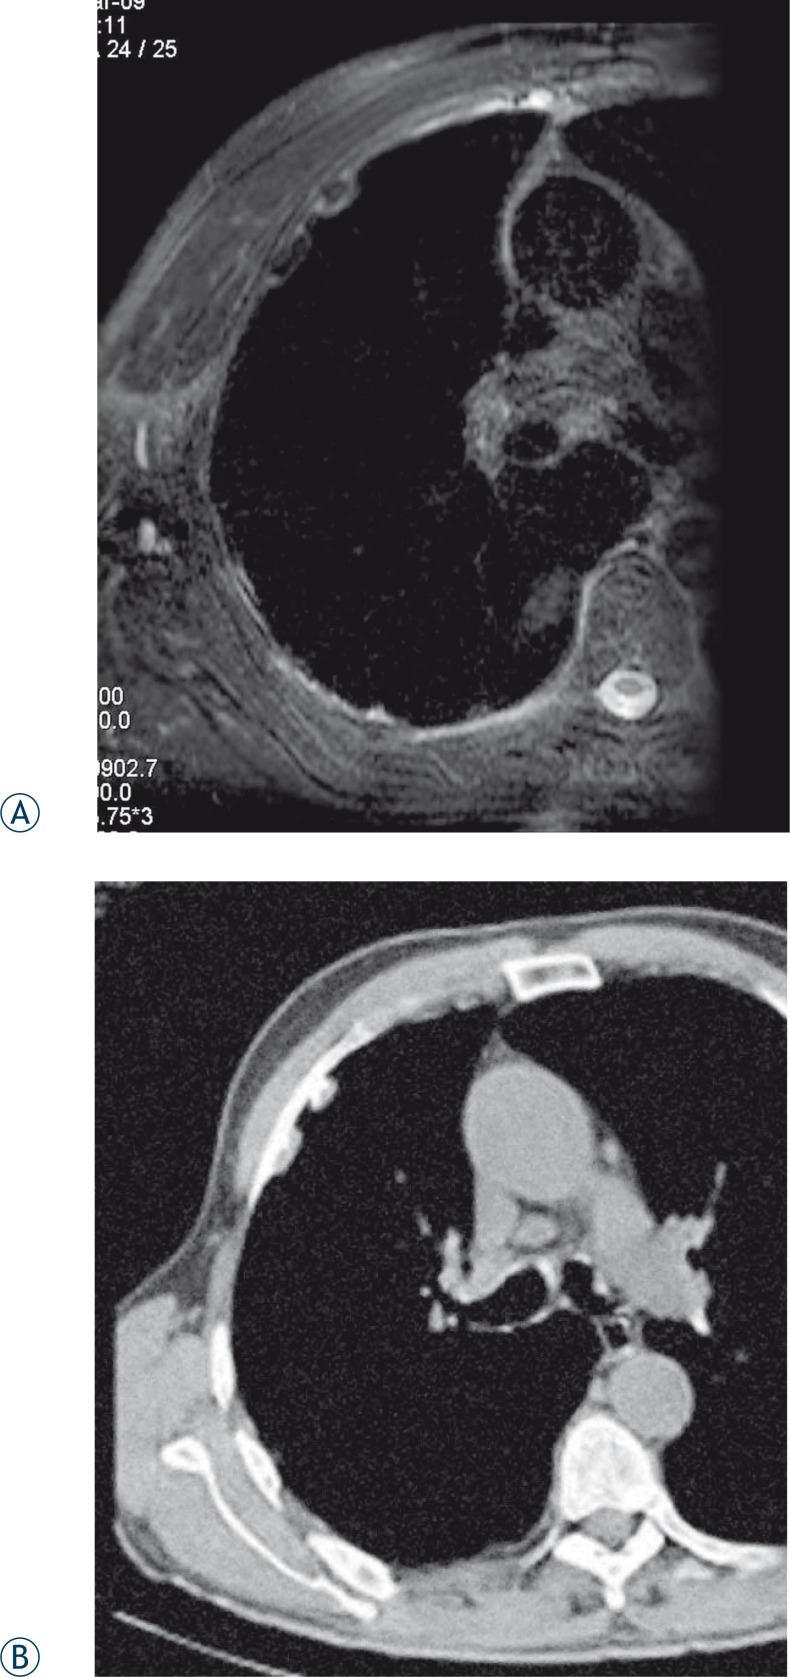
MRI and CT images of the same patient. (A) *T2* fs-weighted MRI image in axial plane shows benign hypo-intense pleural plaques with hyper-intense rim. (B) On CT image calcification on the lateral side of the plaques are more delineated than on MRI images.
